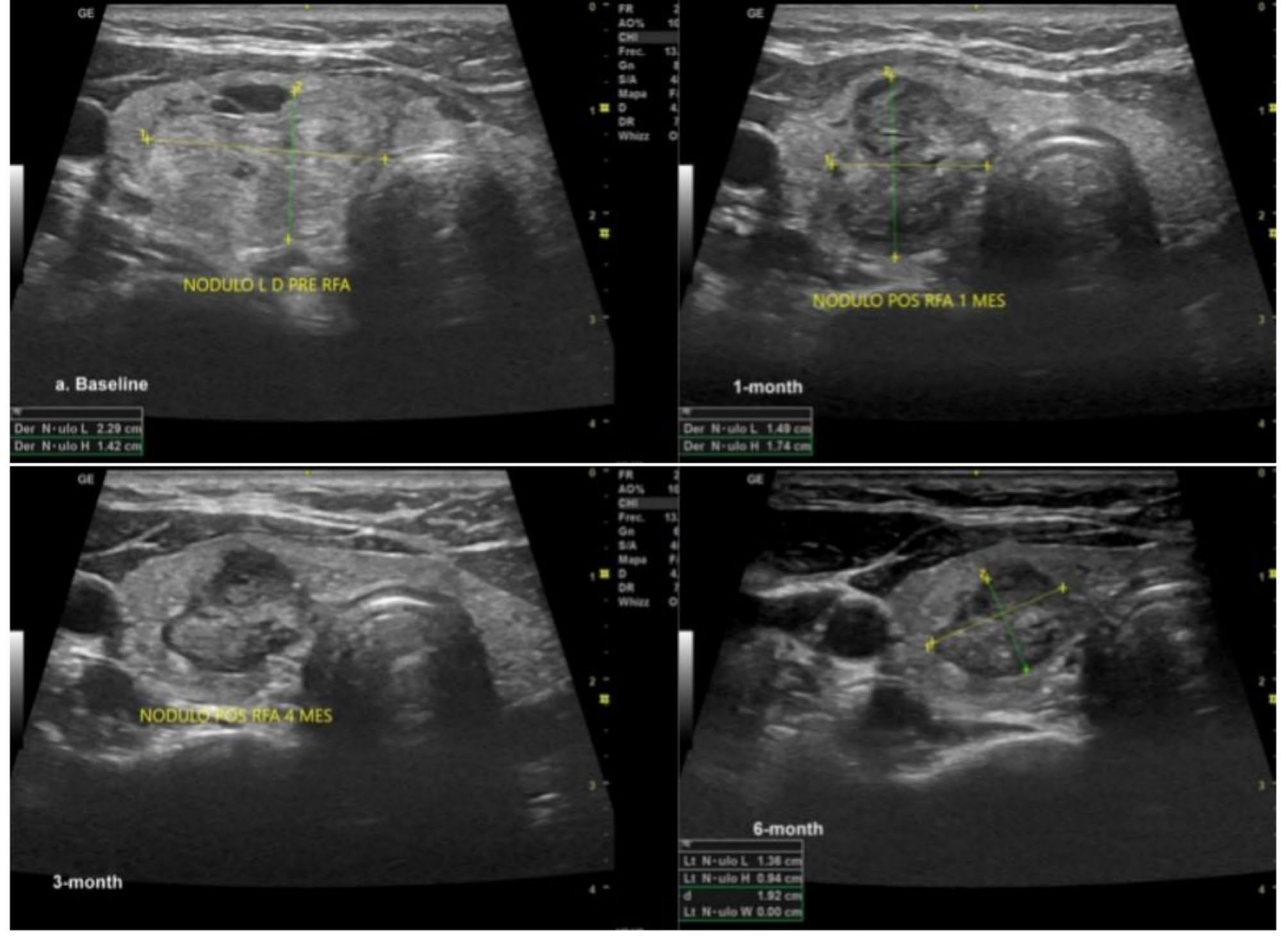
Benign thyroid nodules pre- and post-RFA (1, 3, and 6 months), see the changes in volume and the ultrasound aspect of the nodule due to coagulative necrosis caused by the heat
